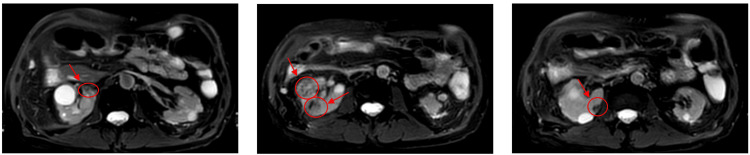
Renal lesions identified on MRI
